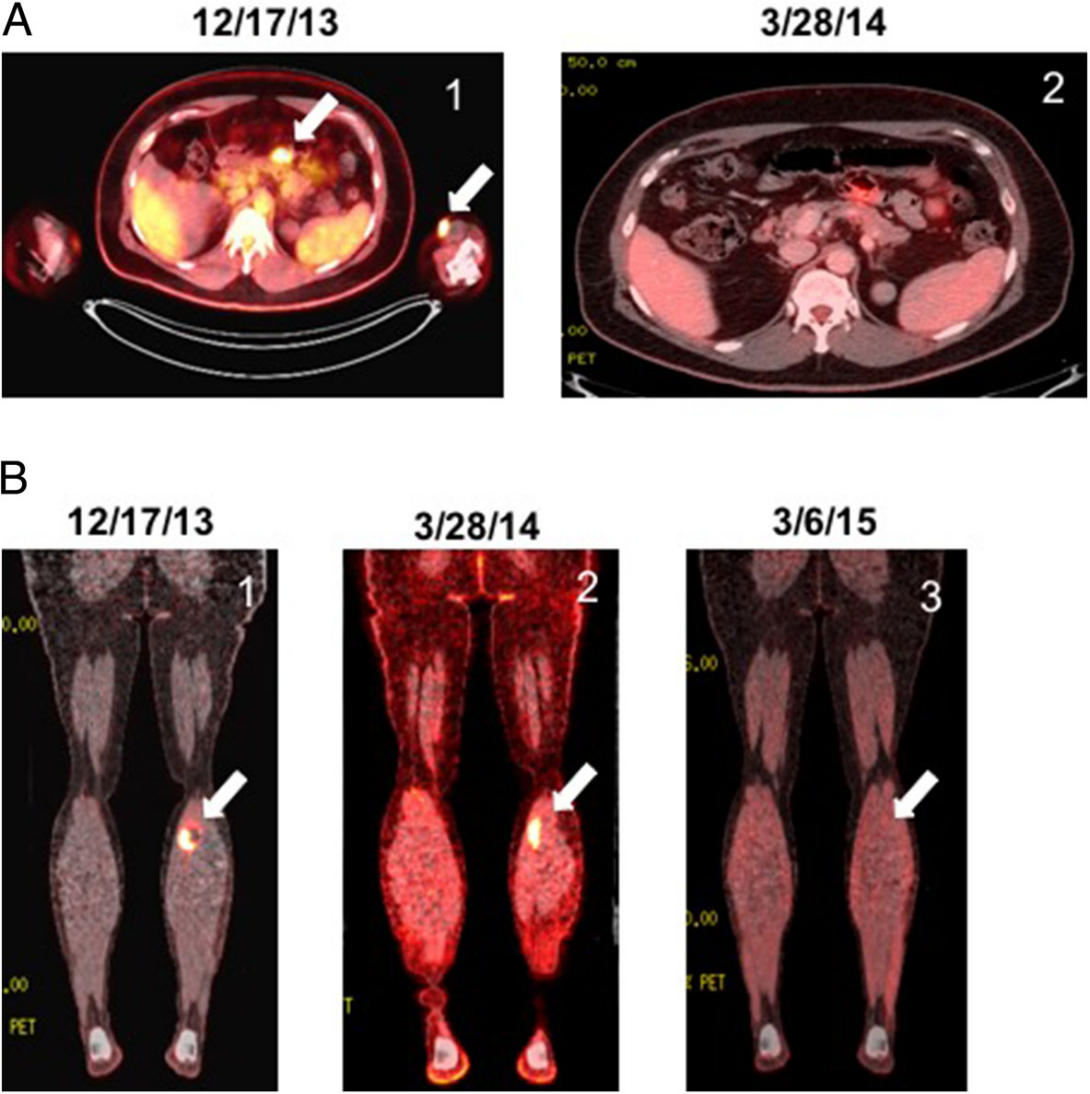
PET CT Imaging of Patient Undergoing Combination Ipilimumab/Nivolumab Immunotherapy. a
